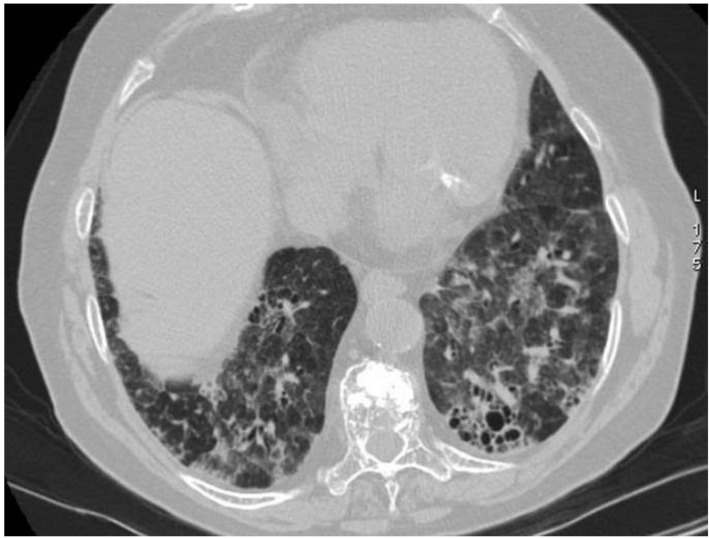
CT scan shows subpleural reticulation and architectural distortion in the lower lobes in an 81-year-old female with AAV. Small, rounded cysts with thick walls are distributed in concentric layers in the subpleural region of the lower left lobe (honeycombing).
